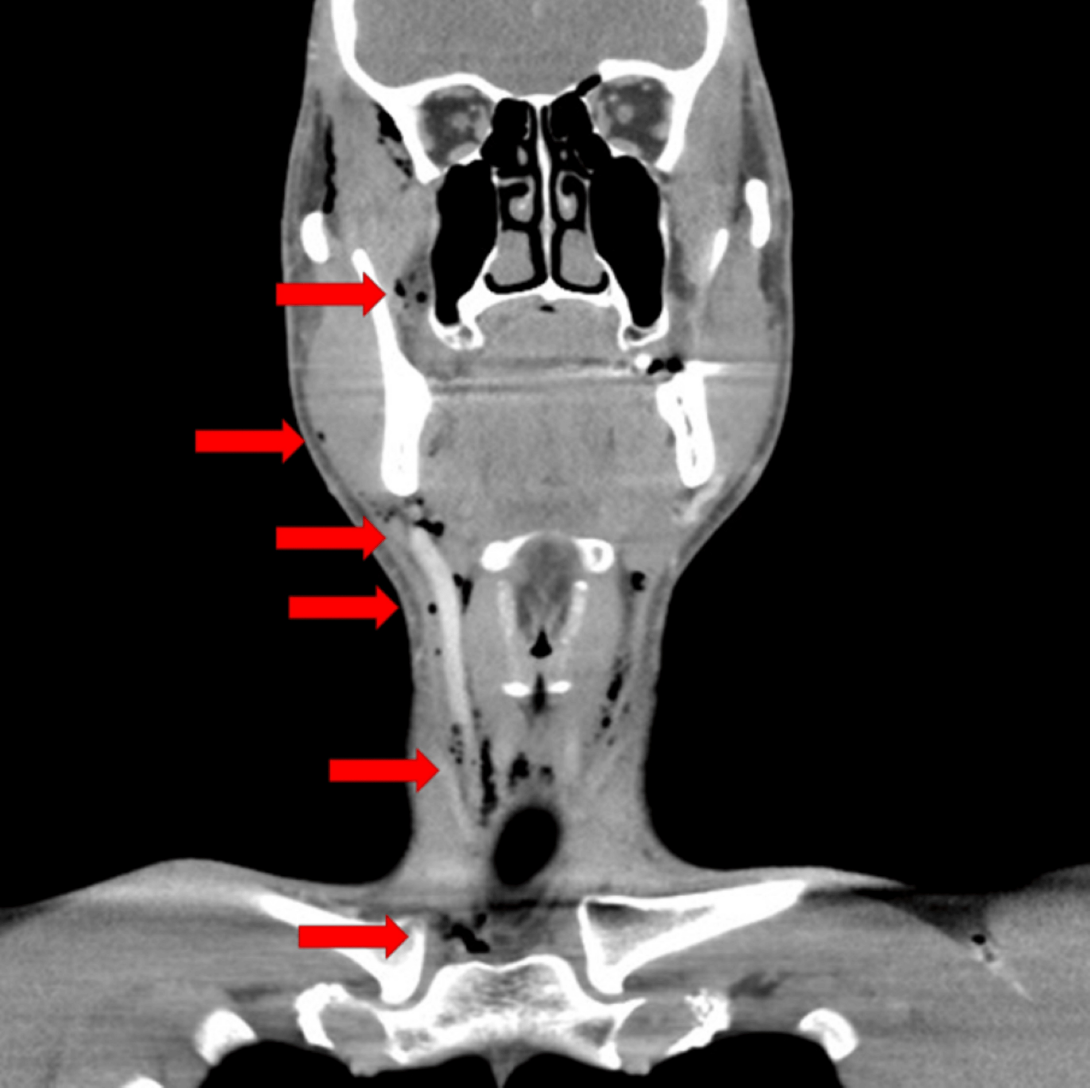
Computed Tomography: Coronal View Red arrows indicate areas of subcutaneous emphysema.
